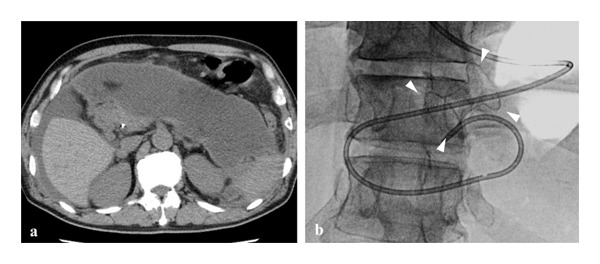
Imaging findings at the time of transfer and initial drainage. (a) Contrast‐enhanced computed tomography at the time of transfer to our institution demonstrating a large walled‐off necrosis extending into the pelvic cavity, compressing the stomach and resulting in gastric outlet obstruction. (b) Fluoroscopic image demonstrating the placement of a lumen‐apposing metal stent (Hot AXIOS, 15‐mm diameter × 10‐mm length) between the stomach and the WON cavity under endoscopic ultrasonography guidance (arrowheads).
